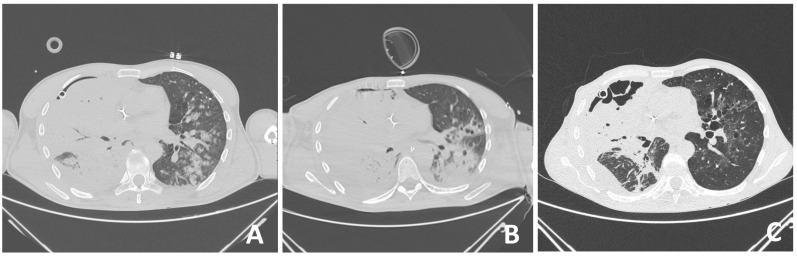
Changes in the lung window of CT in a 53-year-old shipyard worker after severe chest trauma with V/Q mismatch and respiratory failure requiring mechanical ventilation: on admission (A), after 6 days when pneumonia also developed on the left side (B), and after *Stenotrophomonas maltophilia* was identified and treated with sulfamethoxazole/trimethoprim, a regression of lung infiltrates was observed (C).
